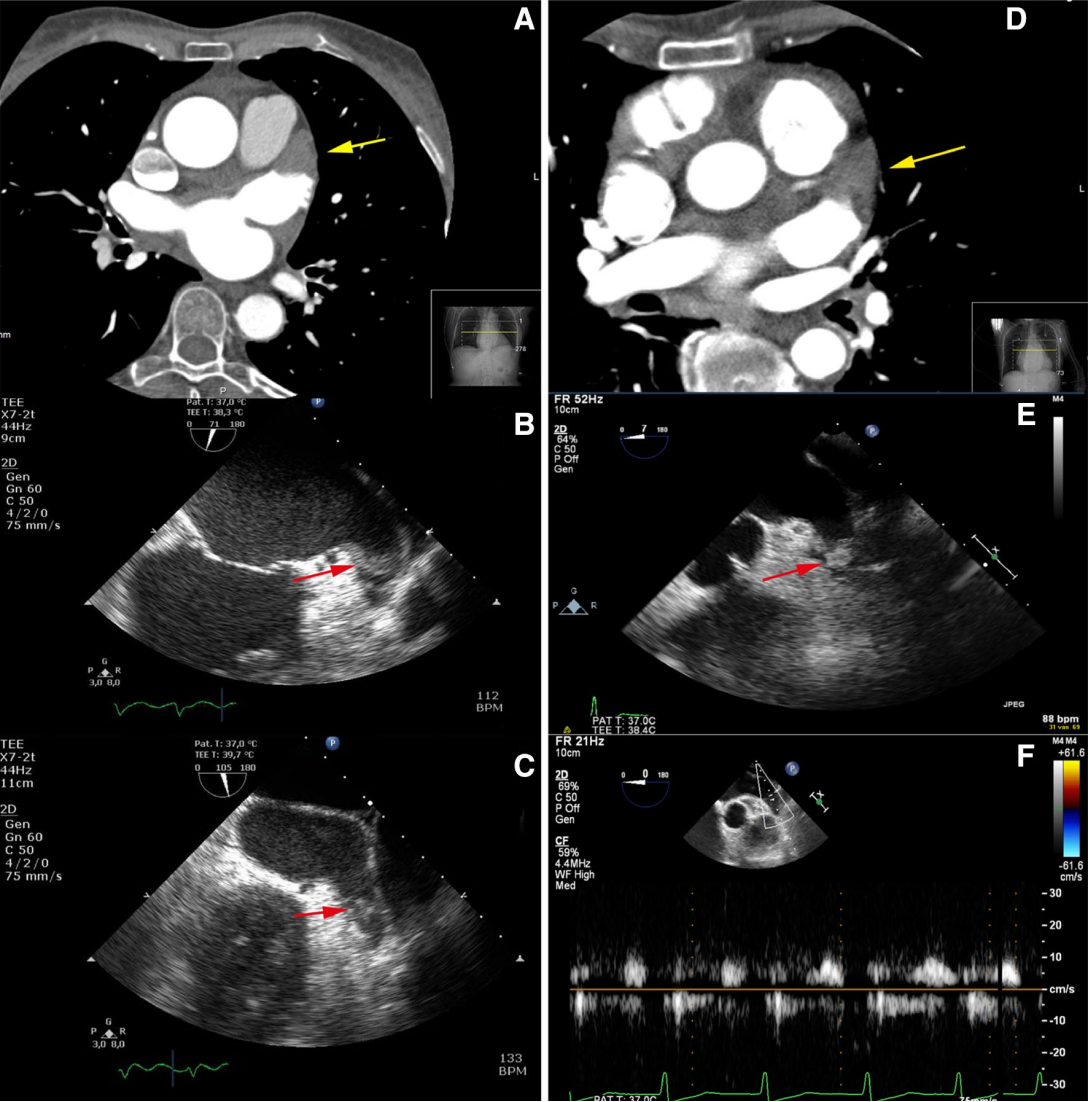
Two patients with a LAA filling defect on CTA based on thrombus. a CTA in axial view showing a LAA filling defect (*yellow arrow*). The area of low attenuation shows a homogeneous aspect, HU 50–70 and a well-defined concave border. b, c TEE showing a solid structure adherent to the LAA wall most likely thrombus (*red arrow*). d CTA in axial view showing LAA filling defect (*yellow arrow*). The area of low attenuation shows a homogeneous aspect, HU 70–90 and a well-defined convex border. e TEE showing LAA thrombus (*red arrow*). f Pulsed-wave Doppler showing low LAA flow velocity
